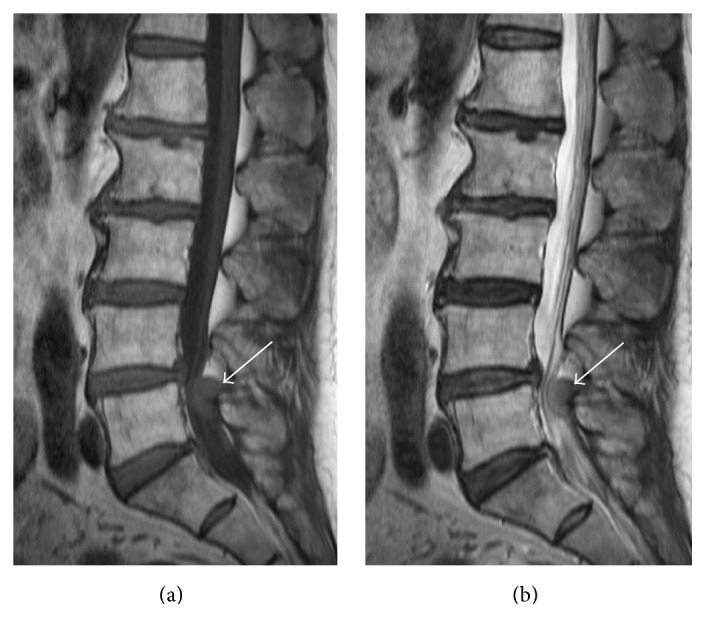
Sagittal T1-weighted MR image (a) and sagittal T2-weighted MR image reveal solitary spinal epidural mass (arrow) showing slightly high signal intensity as compared with signal intensity of skeletal muscle.
